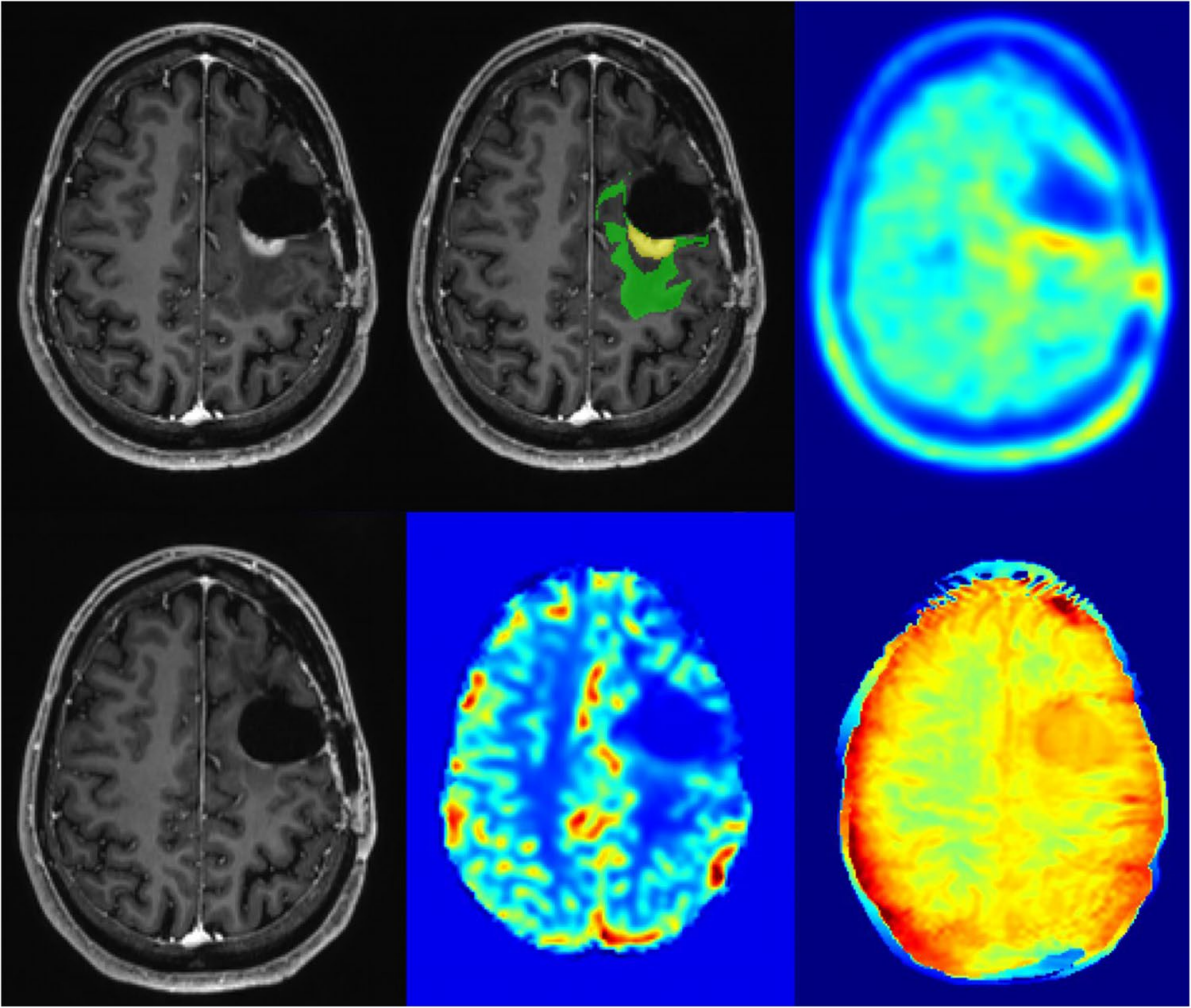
Example images of TRC in a 34-year-old male with a new contrast enhancing focus next to the resection cavity after therapy of a left frontal GBM (upper left: ce T1-w, lower left: previous time point, upper middle: automated segmentation overlay (green: new FLAIR edema area; yellow: new ce area), lower middle: CBV, upper right: [^18^F]-FET-PET, lower right APTw)
